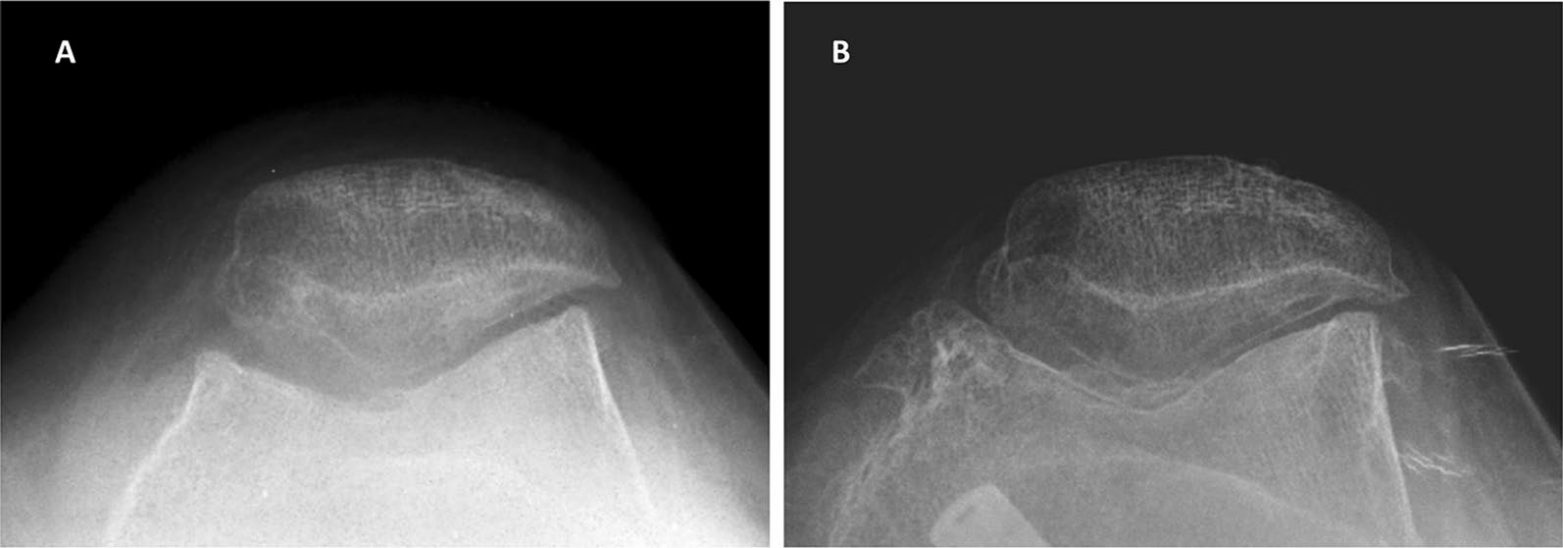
Merchant view radiographs of a 58-year-old woman (case 1). A Preoperative radiograph showing Kellgren–Lawrence grade 2 patellofemoral joint osteoarthritis. B Follow-up radiograph 5 years after surgery showing Kellgren–Lawrence grade 4 patellofemoral joint osteoarthritis
